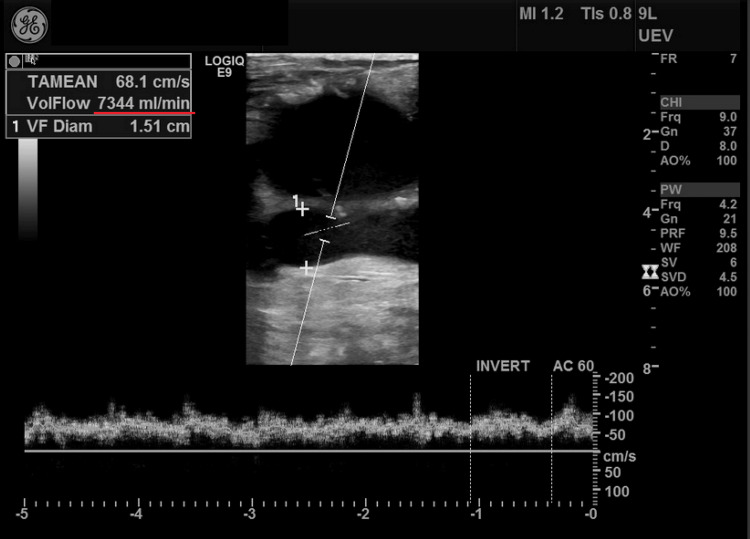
High-flow arteriovenous fistula in ultrasonography (flow rate underlined).
